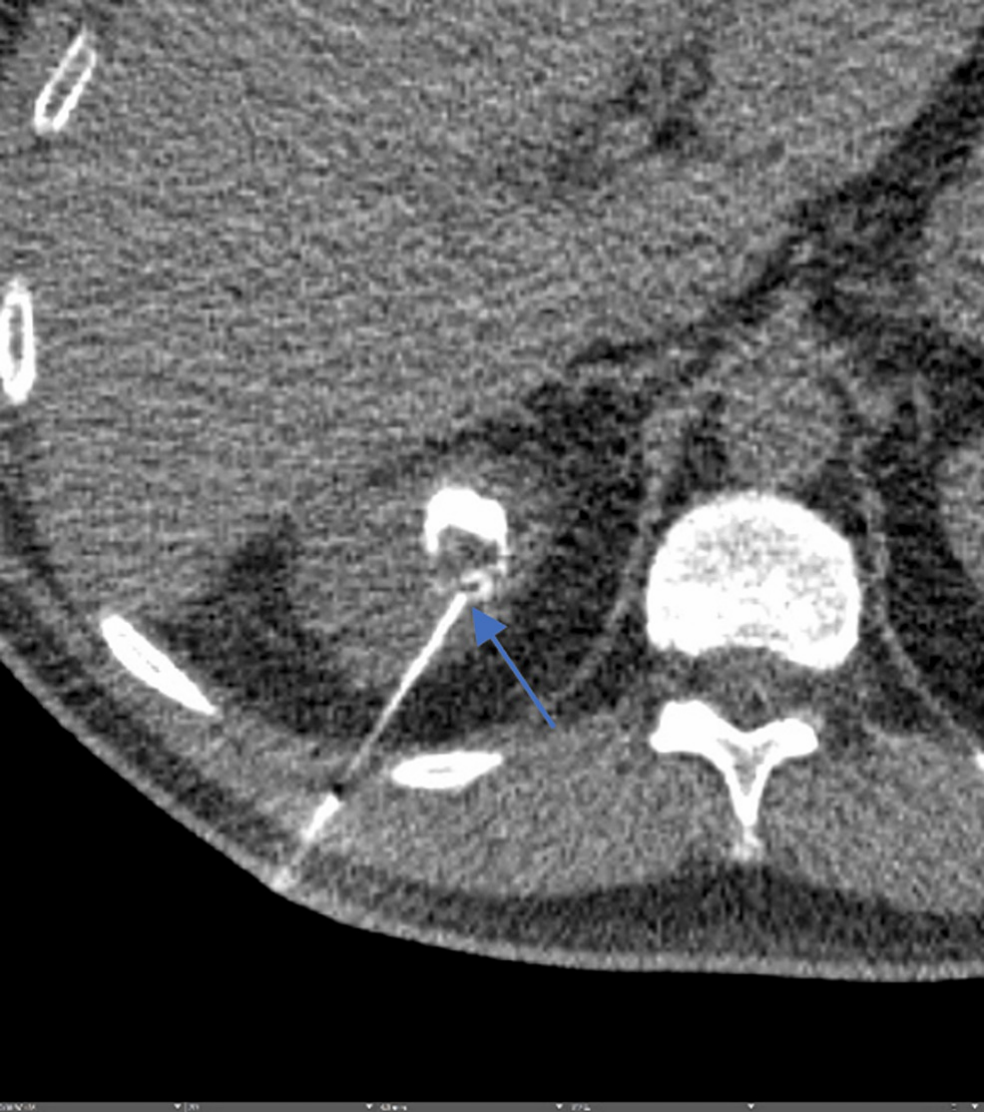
CT-guided aspiration. Arrow: Needle inside the cyst with contrast injected into the cyst cavity and small amount of debris/hemorrhage within the cyst
